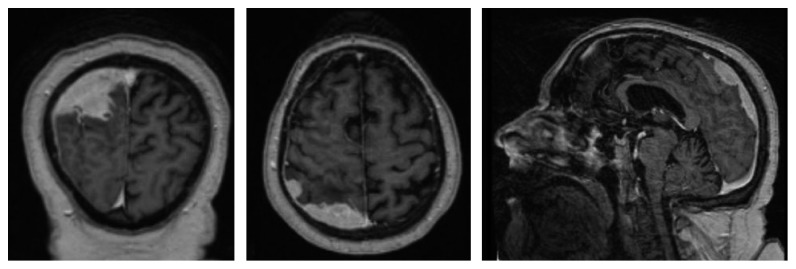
MRI showing right solid extra-axial parieto-occipital lesion with typical characteristics of meningeal myelomatosis. The tumor adapts to the underlying brain surface, is intensely enhanced with contrast, and shows adjacent pachymeningeal enhancement with focal spread through leptomeninges towards sulci in the proximal convexity, associated with vasogenic edema but not causing intracranial herniation.
